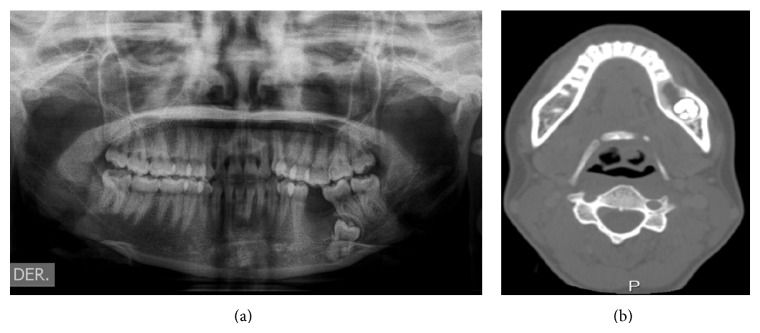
Panoramic radiograph (a) and axial CT scan (b) revealing an ill-defined radiolucent lesion around an impacted mandibular left first molar.
